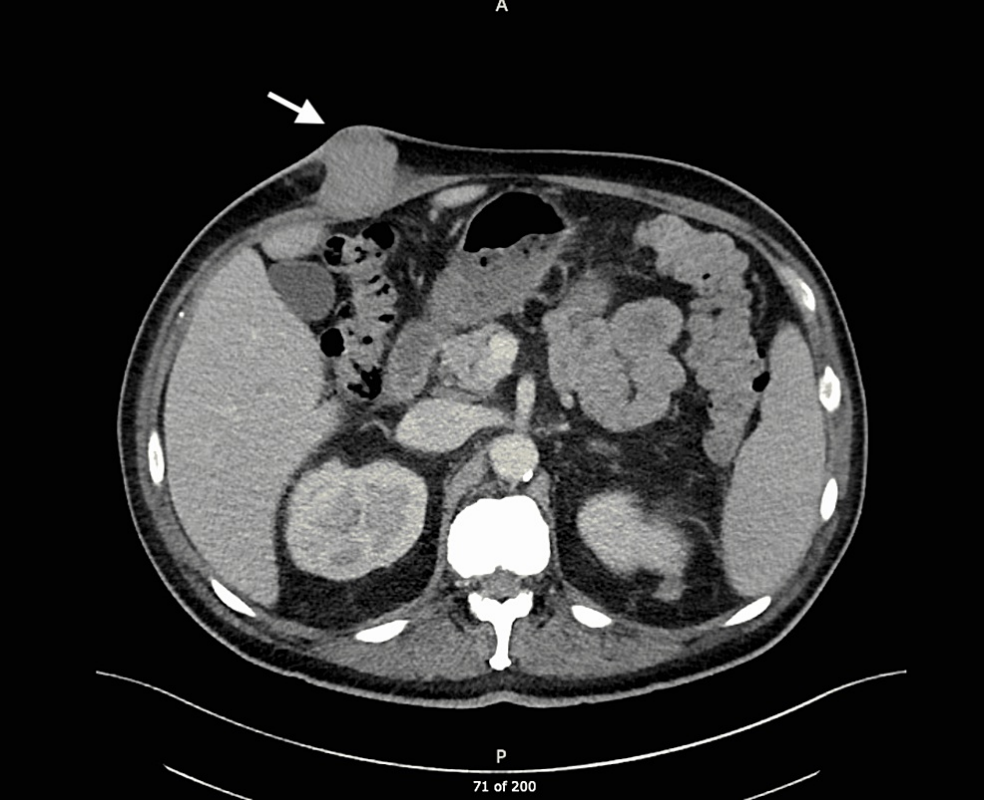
Axial CT image showing dominant lesion (arrow) in the right upper abdominal wall.
